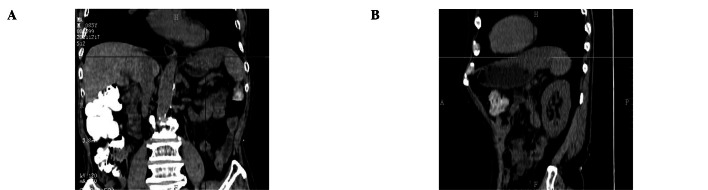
Four months subsequent to GIST resection. (A) Coronal and (B) sagittal reconstructed CT images revealing a heterogeneous mass, with a maximum diameter of 8.3 cm and a density of −24 to 35 HU, situated between the gastric fundus and spleen. The stomach wall and the upper pole of the spleen appeared to have been invaded. GIST, gastrointestinal stromal tumor; CT, computed tomography.
